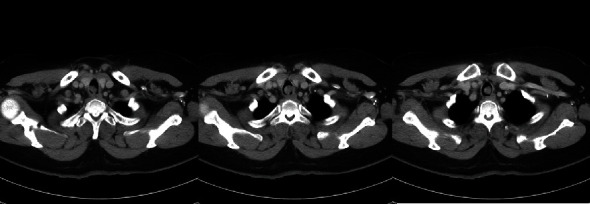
Transverse computed tomography images of the upper thorax showing upper airway obstruction due to a tracheal schwannoma.
